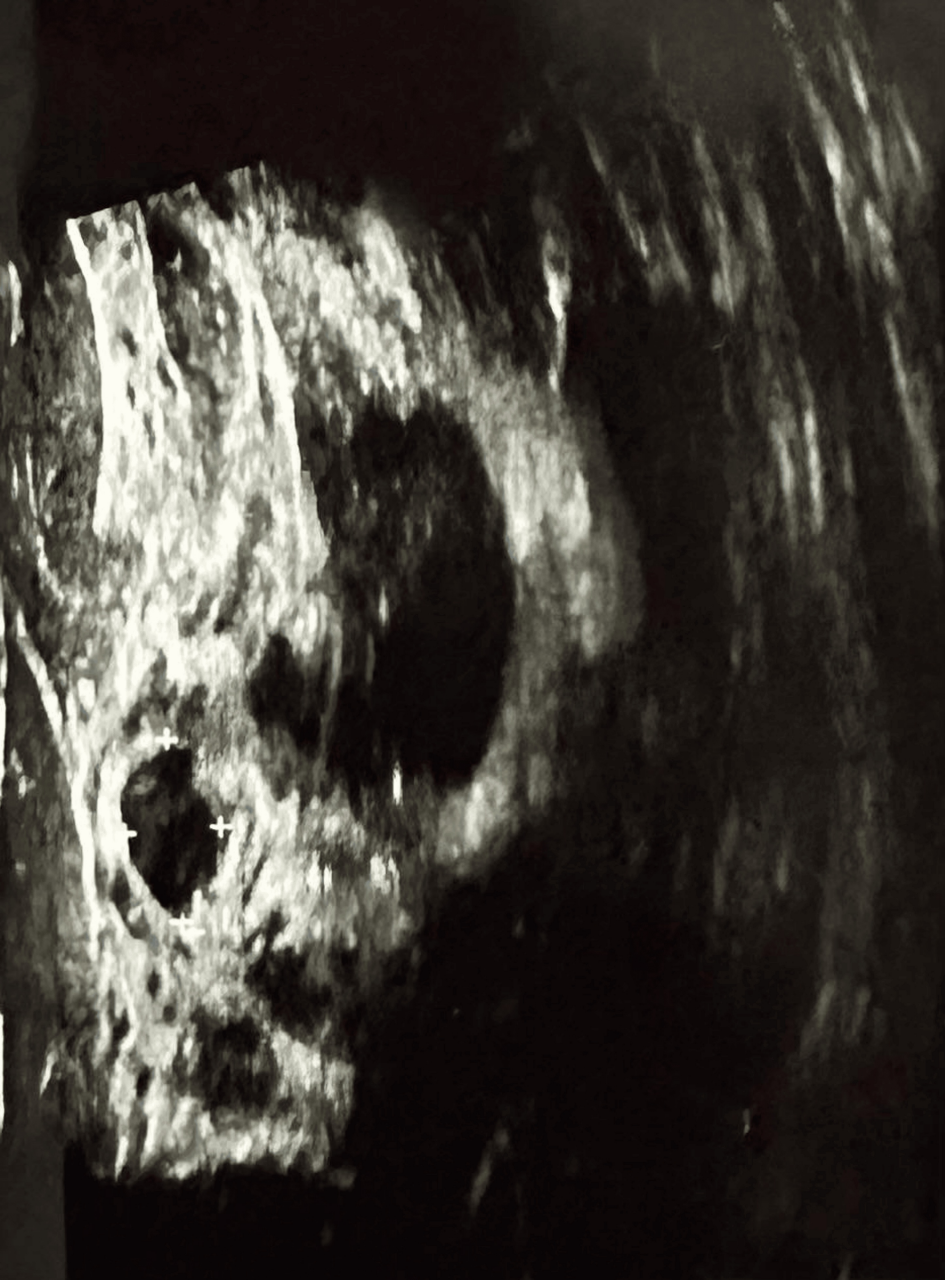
Ultrasonography findings: hypoechoic lesion of the right breast
